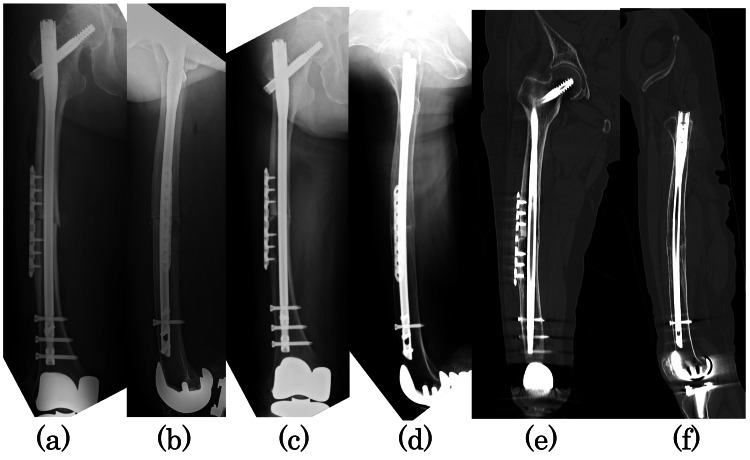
Radiographs taken immediately after surgery and at one year and two months postoperatively Immediate postoperative radiographs: (a) anteroposterior and (b) lateral images. Radiographs at one year and two months postoperatively: (c) anteroposterior and (d) lateral images. Plain computed tomography at one year and two months postoperatively: (e) coronal and (f) sagittal images. Anterior, posterior, and medial bony fusion of the osteotomy has been achieved, and bony bridging has been achieved on the lateral side.
